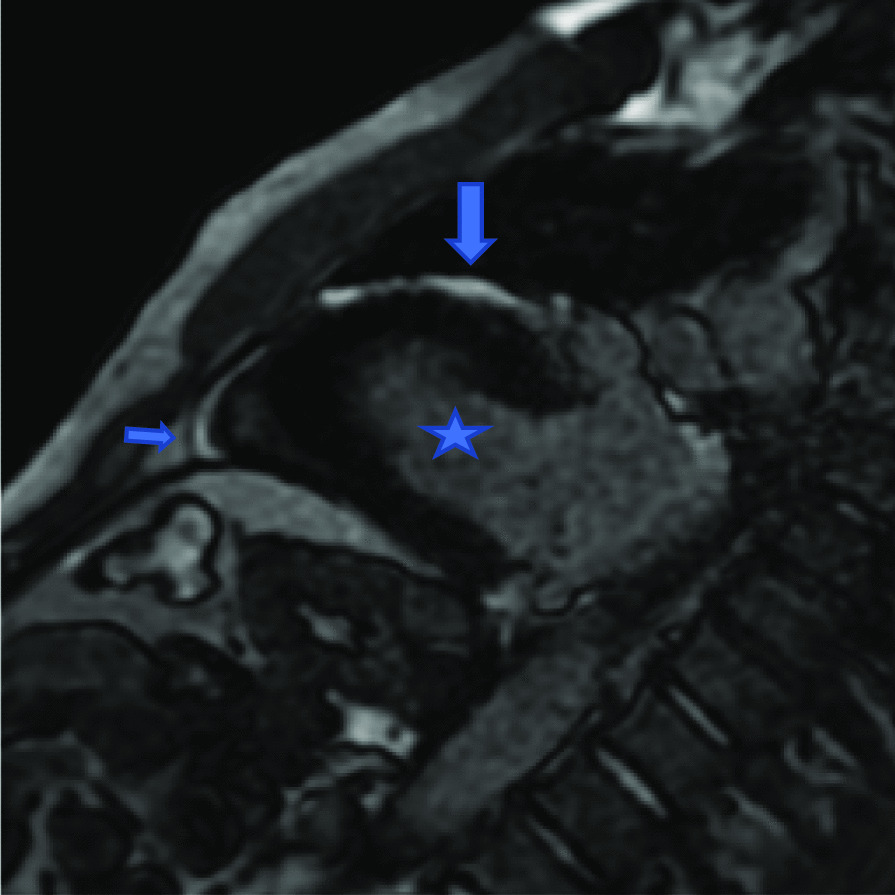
Cardiovascular magnetic resonance imaging, fast gradient echo, two-chamber view showing late gadolinium enhancement of the pericardium over the left ventricular anterior wall (arrow) and a trivial pericardial effusion (small arrow). Star denotes left ventricular cavity. Star denotes the left ventricle
